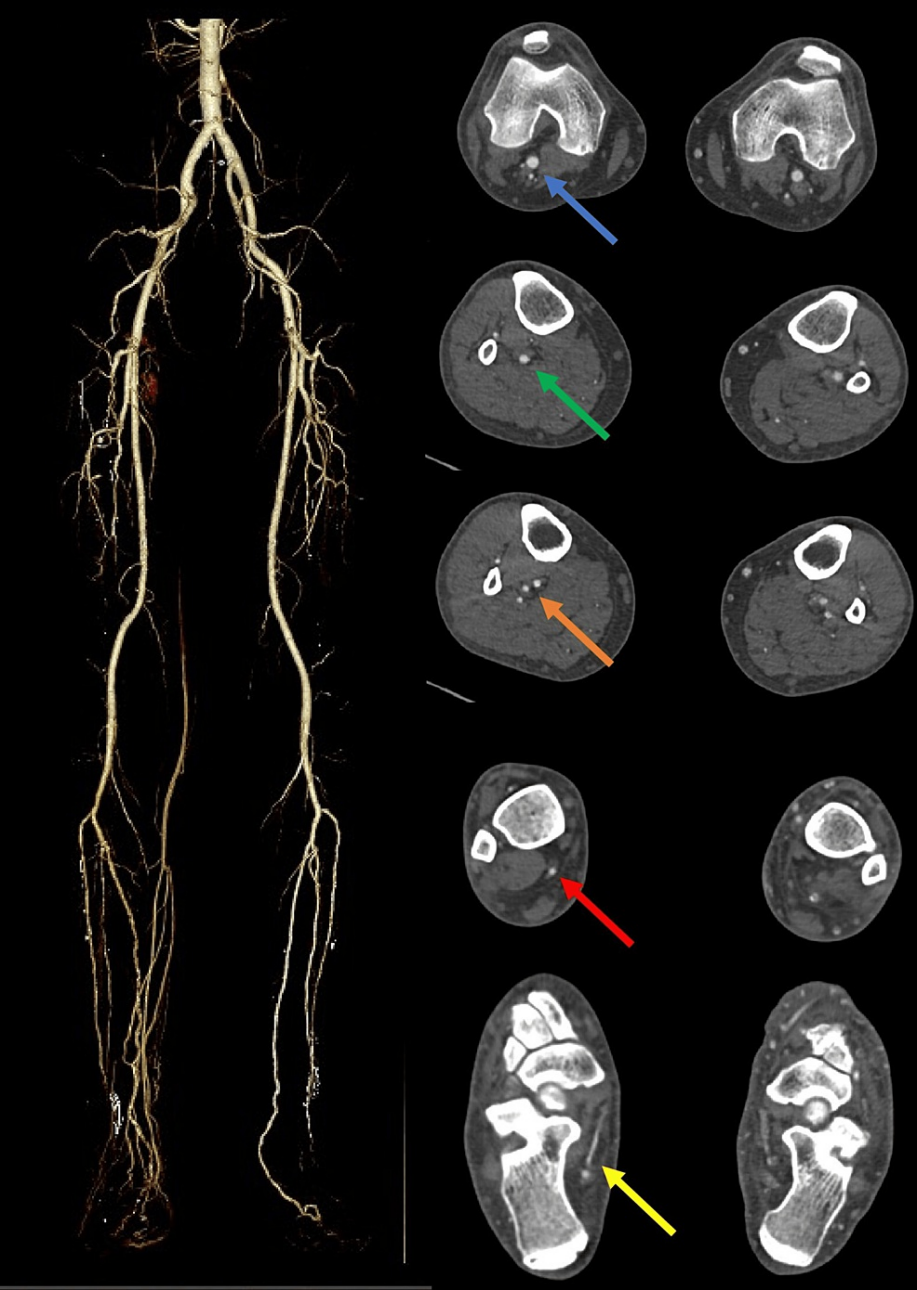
Example of lower limb CTA with arteries labeled at each level CTA: computed tomography angiography Blue arrow - popliteal artery; green arrow - tibioperoneal trunk; orange arrow - posterior tibial artery and peroneal artery; red arrow - posterior tibial artery; yellow arrow - medial plantar artery.
